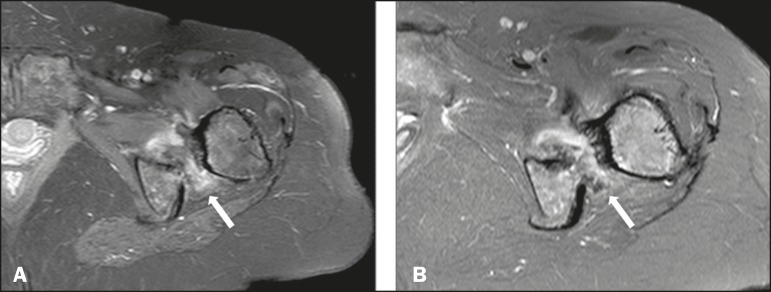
A: Contrast-enhanced T1-weighted magnetic resonance imaging scan with fat
